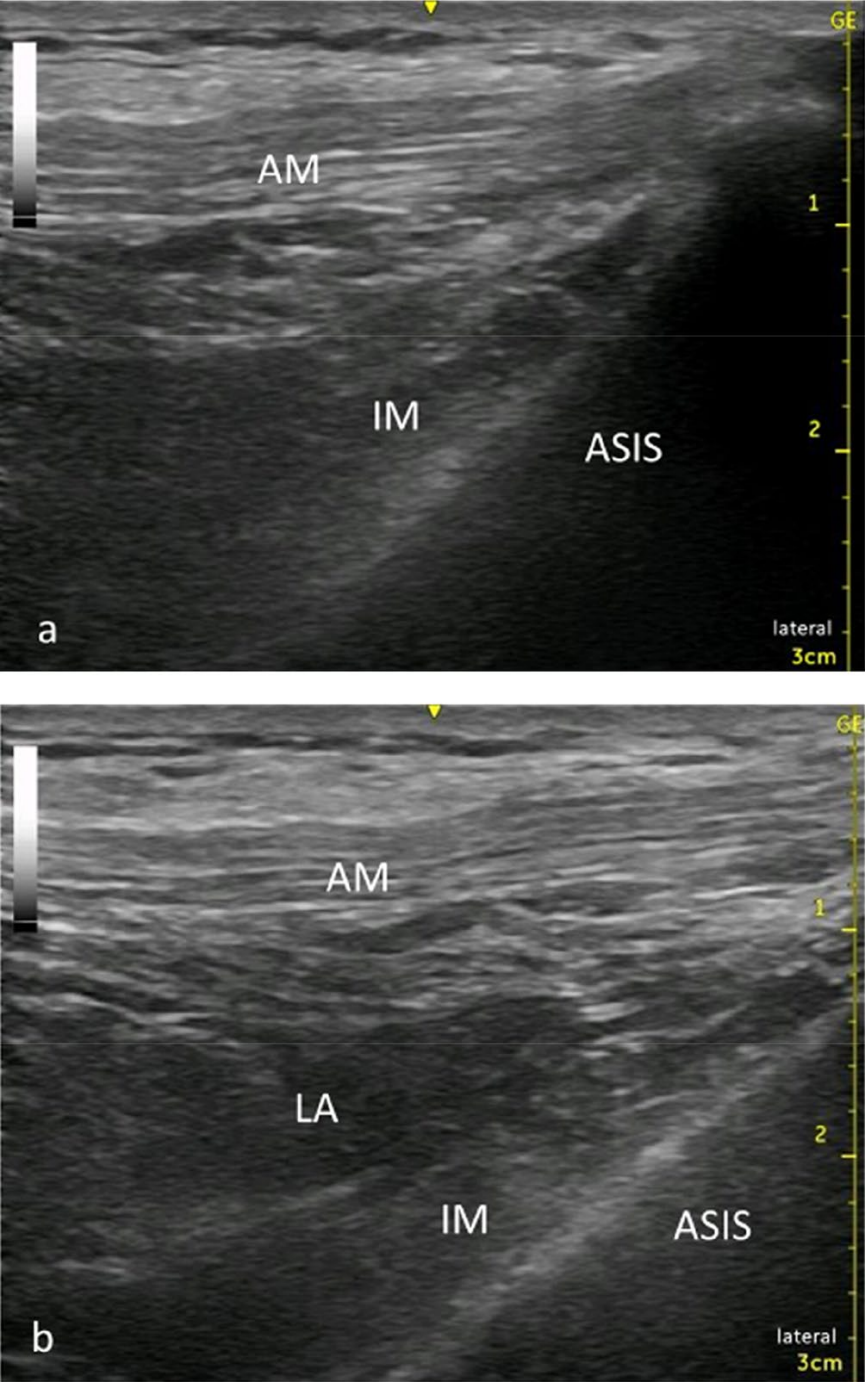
Ultrasound image of pre injection (a), ultrasound image of post injection (b). *ASIS* Anterior superior iliac spine, *IM* iliacus muscle, *AM* abdominal muscles, *LA* local anesthetic.
